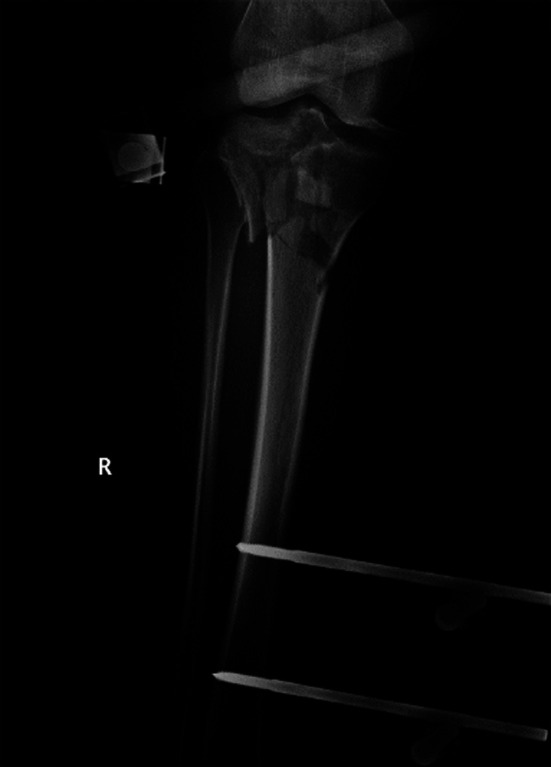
X-ray film of tibial plateau fracture before operation.
